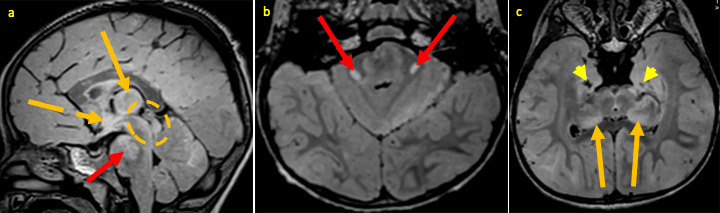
30-month-old male with rAHNE. Brain MRI obtained during his second presentation for AHNE: sagittal (a) and axial (a, c) FLAIR images demonstrating new hyperintense signal abnormalities in the subthalamic area [broken orange arrow, (a)], pons extending into middle cerebellar peduncles [red arrows (a & b)], and midbrain [broken orange circle (a)] as well as worsening hyperintense signal abnormalities (orange arrows) in the thalami (a & c). Note areas of evolving encephalomalacia (yellow arrow heads) in bilateral medial temporal lobes (c). AHNE, acute hemorrhagic necrotizing encephalopathy; FLAIR, fluid attenuation inversion recovery.
